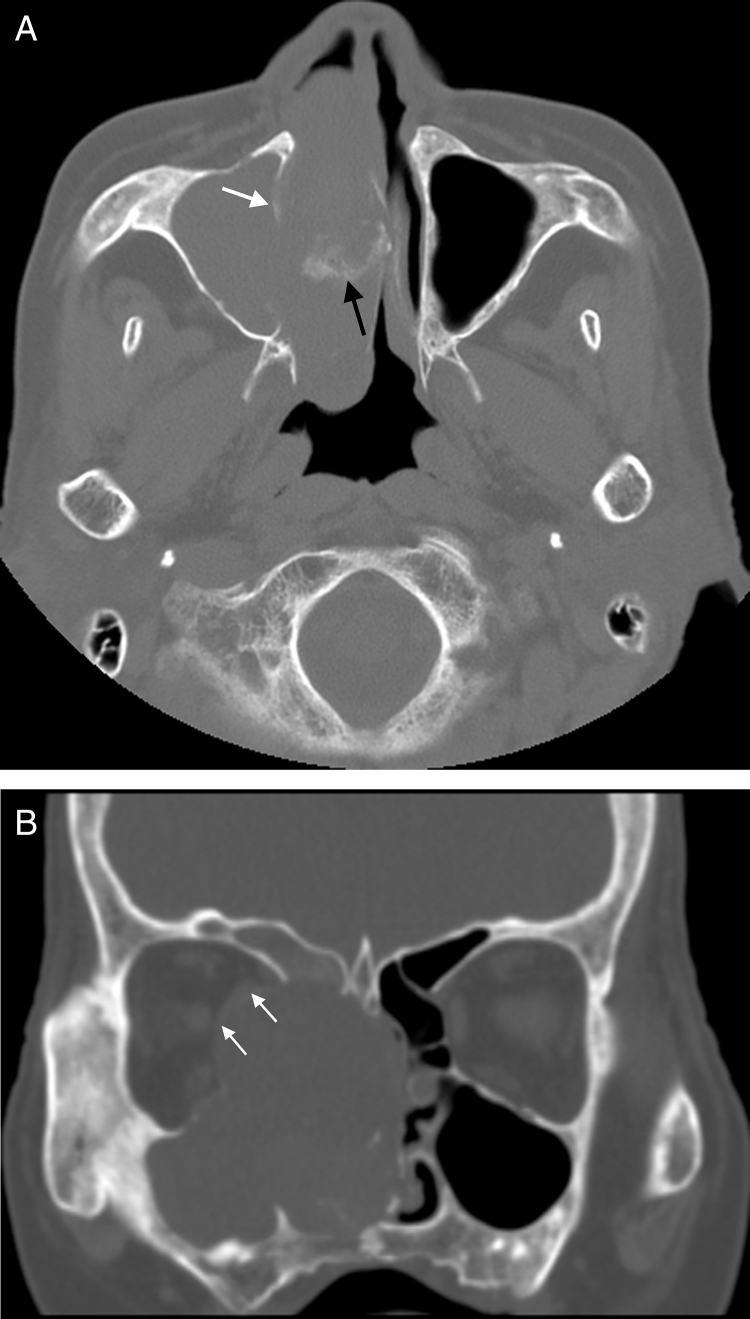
(A) Axial CT scan shows the soft tissue mass in the paranasal sinus and nasal cavity. Bone destruction of the medial wall of maxillary sinus is apparent and the margin is obscure (white arrow in A). Patchy calcification is found in the lesion (black arrow in A). (B) Coronal CT scan shows main body of soft-tissue mass is located in ethmoid sinus and nasal cavity and it damaged bone of right orbit with compression of the medial rectus (white arrow in B). The wall of the right ethmoidal sinus, right maxillary sinus and right frontal sinus were damaged.
